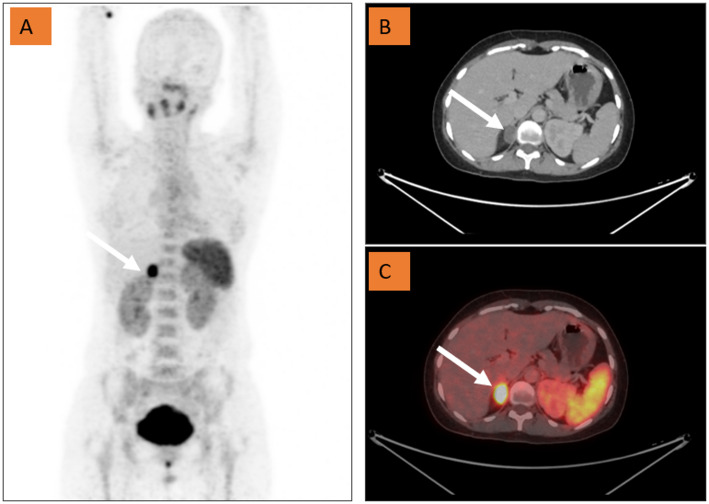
Ga-68 Pentixafor PET-CT (A) Maximum Intensity Projection PET image showing intense uptake in the right suprarenal region. (B-C) Axial CT and fused PET-CT images show increased tracer uptake in the hypodense nodule in the right adrenal gland (arrow), suggestive of a unilateral aldosterone producing adenoma
